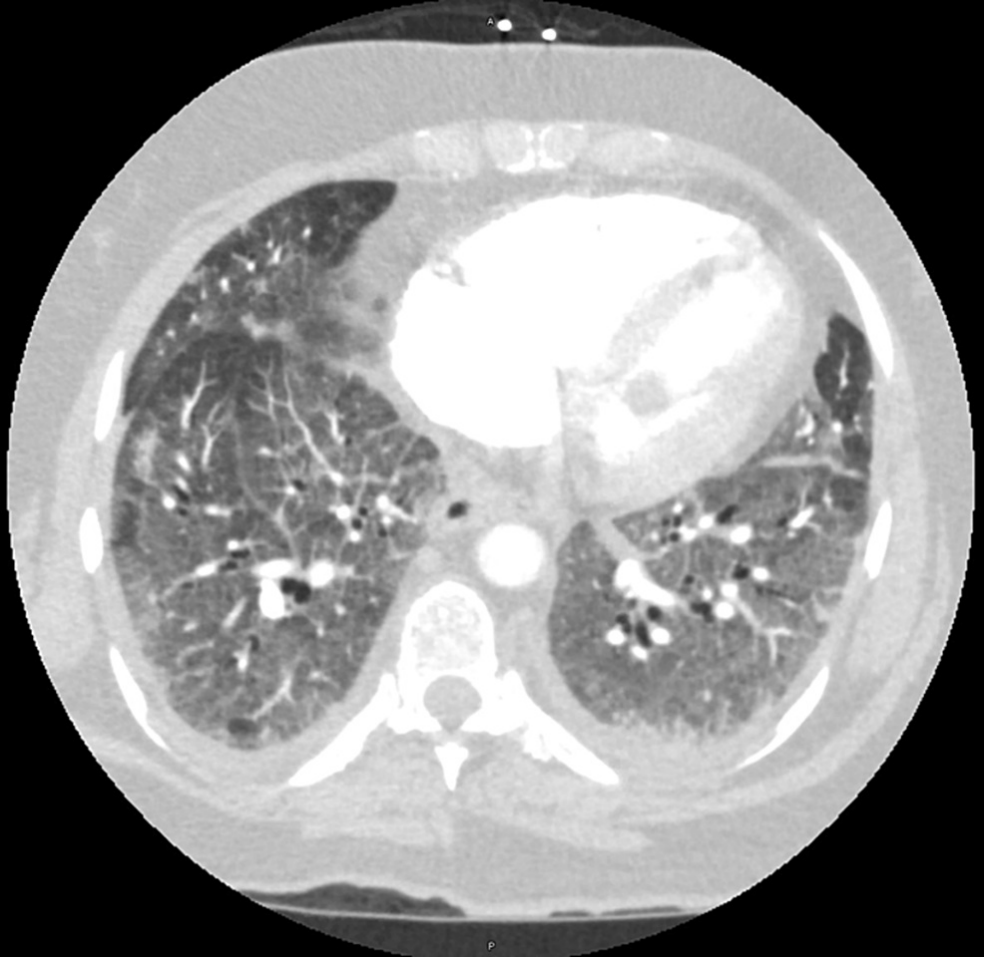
Computed tomography angiogram (CT-A) of the thorax on admission
